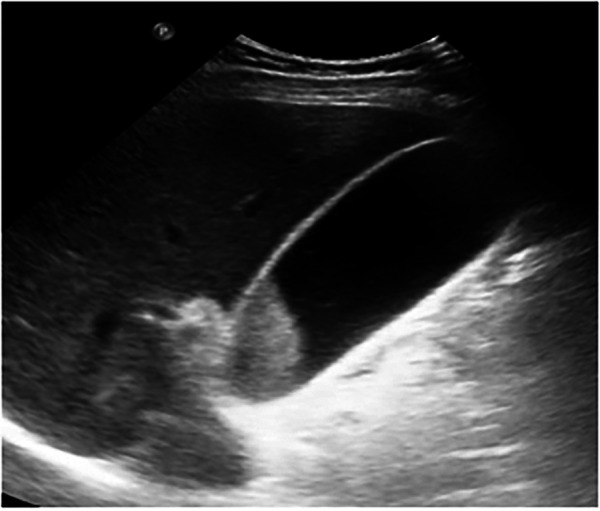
Ultrasound: the gallbladder appears distended with coarse biliary sludge and well-defined walls, without evidence of gallstones or biliary duct dilatation.
